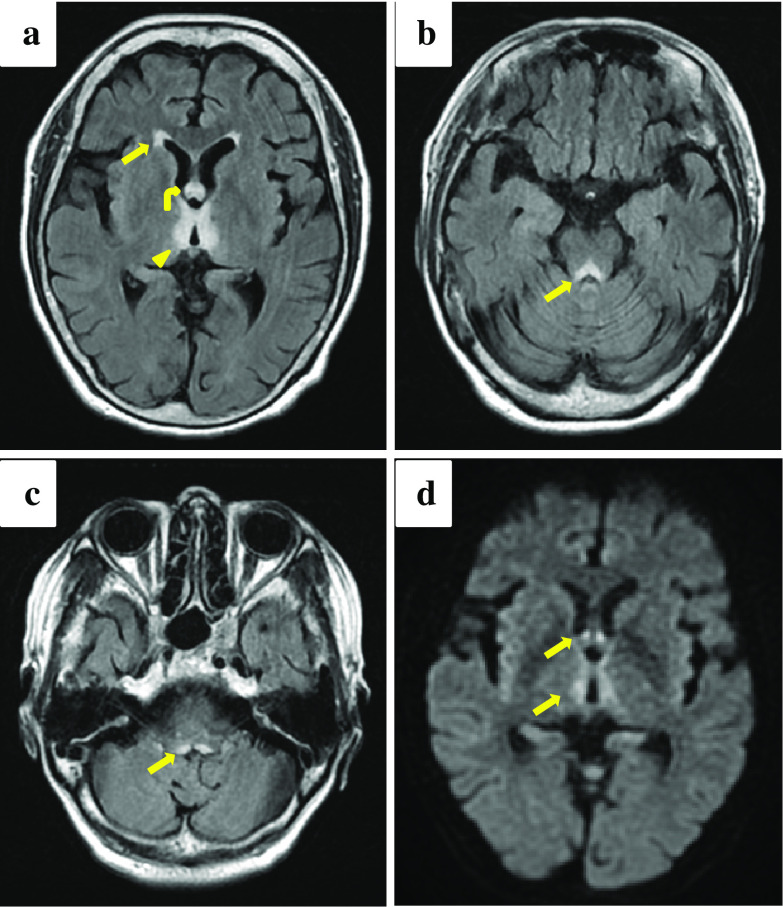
a–d Contrast-enhanced MRI of the head showing symmetrically abnormal hyperintense signal on FLAIR in the frontal horn of lateral ventricle (a, arrow), the medial thalami bordering the third ventricle (a, arrow head), the mammillary bodies (a, curved arrow), the periaqueductal region of the midbrain (b, arrow), and the bottom of the 4th ventricle (c, arrow). DWI imaging showing restricted diffusion corresponding to the FLAIR hyperintensities (d, arrows)
